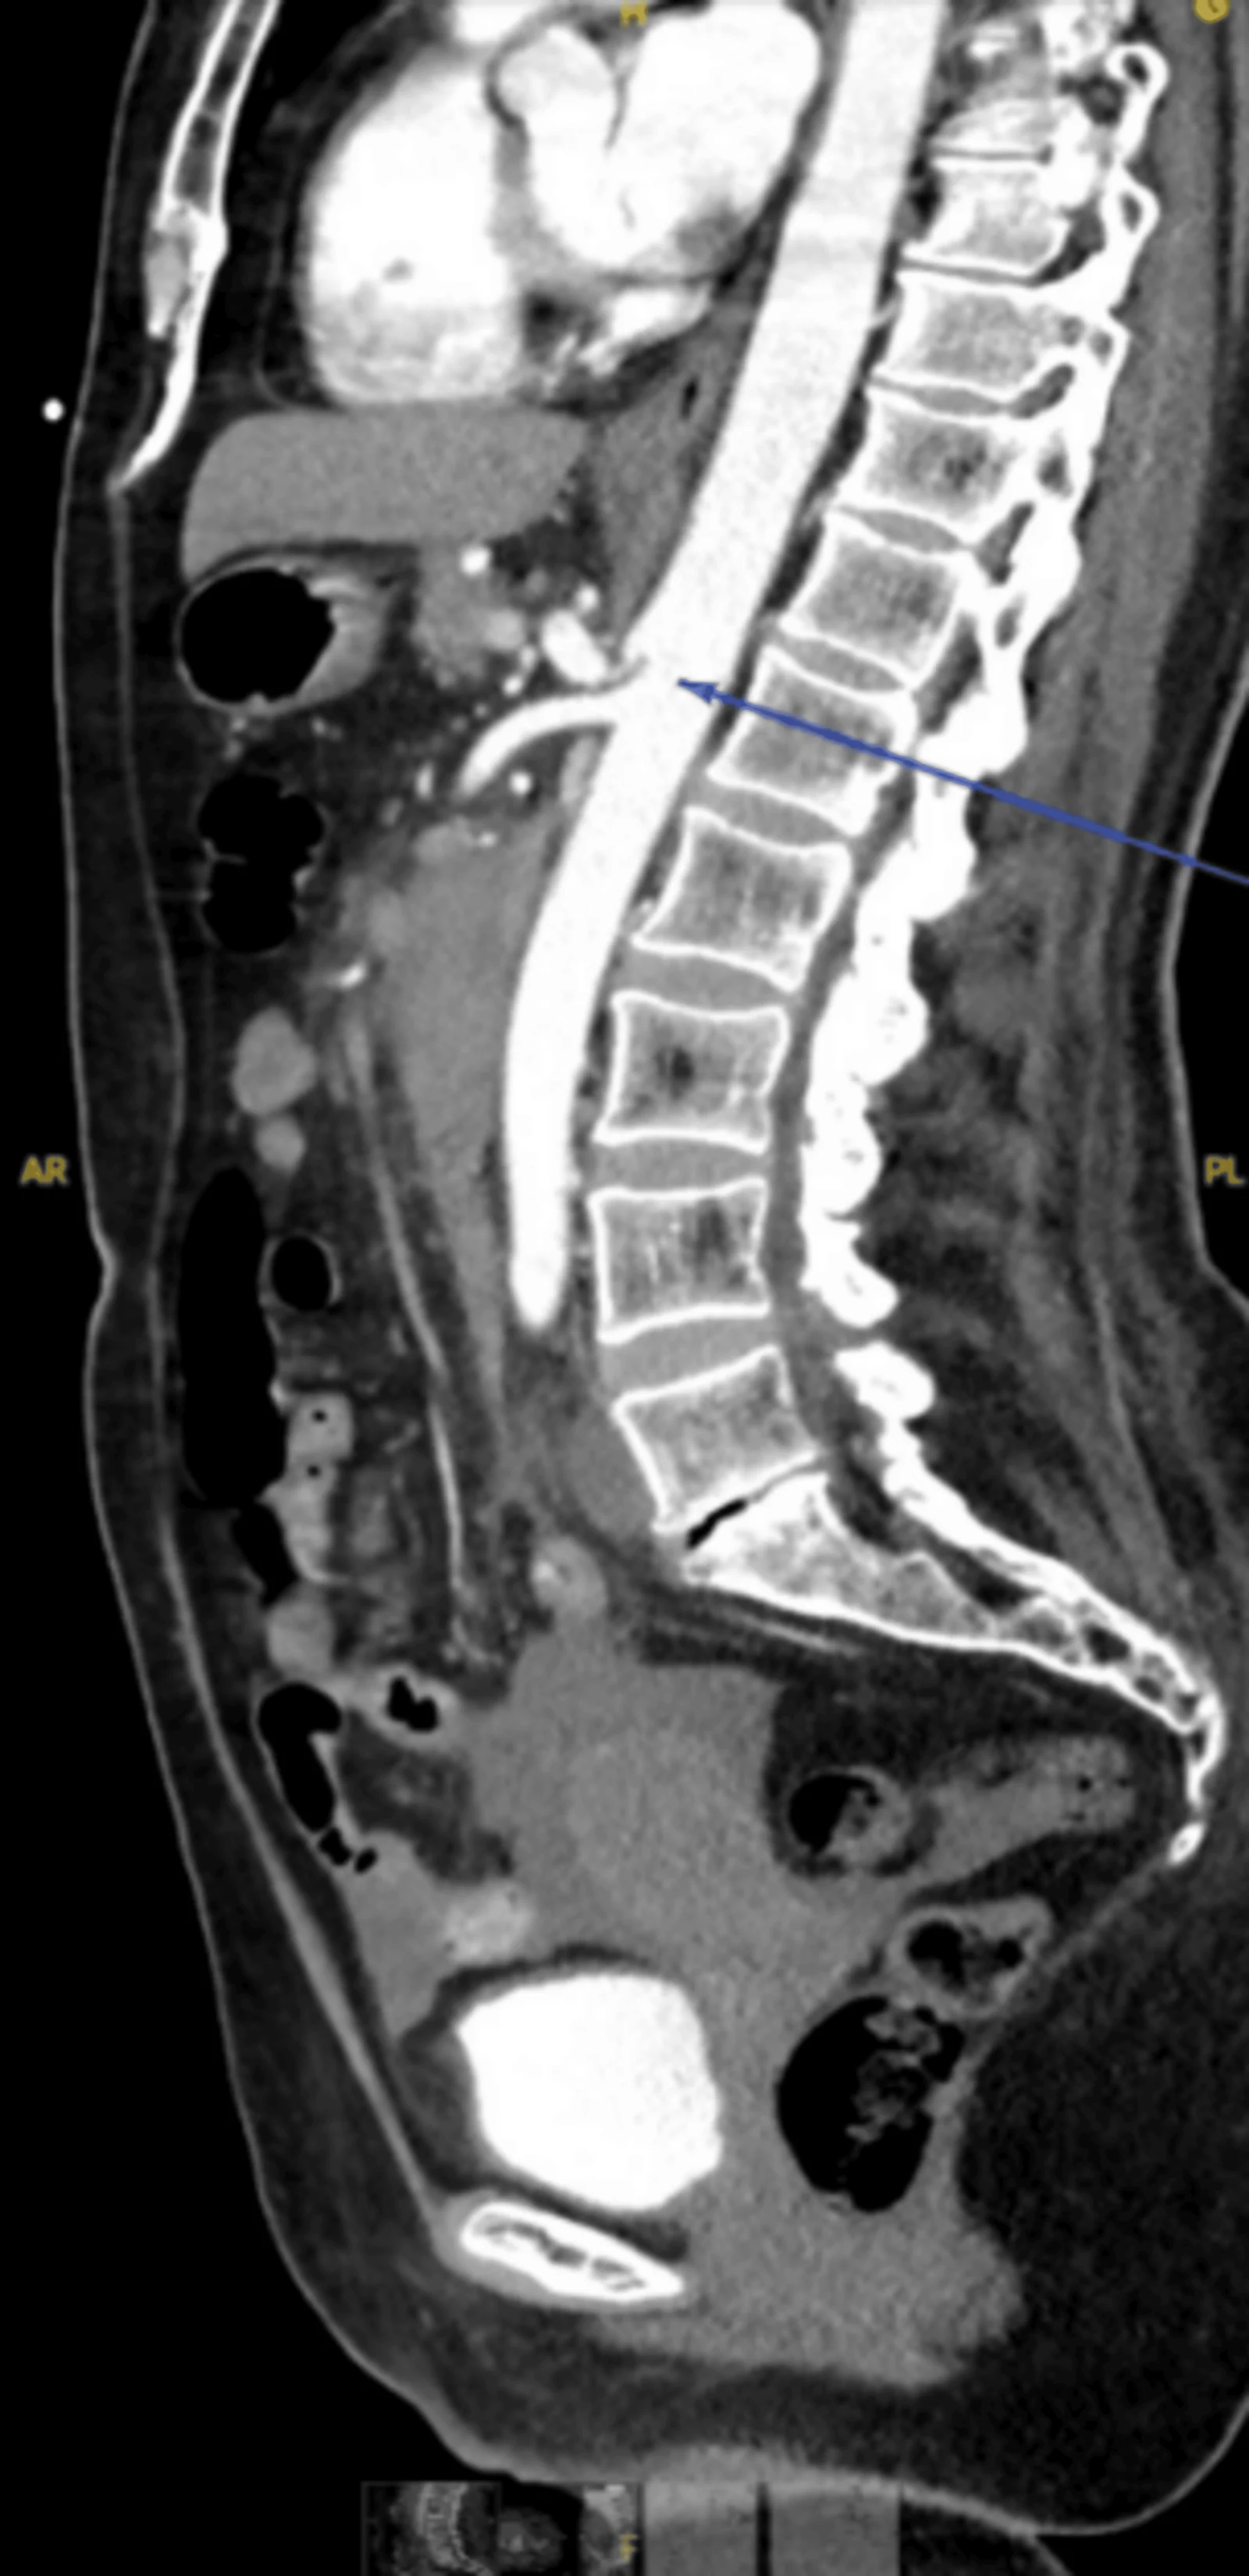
Sagittal view of the CTA revealed severe focal narrowing with a hook-shaped configuration of the proximal celiac artery (blue arrow), supporting the diagnosis of MALS. CTA: Computed tomography angiography, MALS: Median arcuate ligament syndrome
